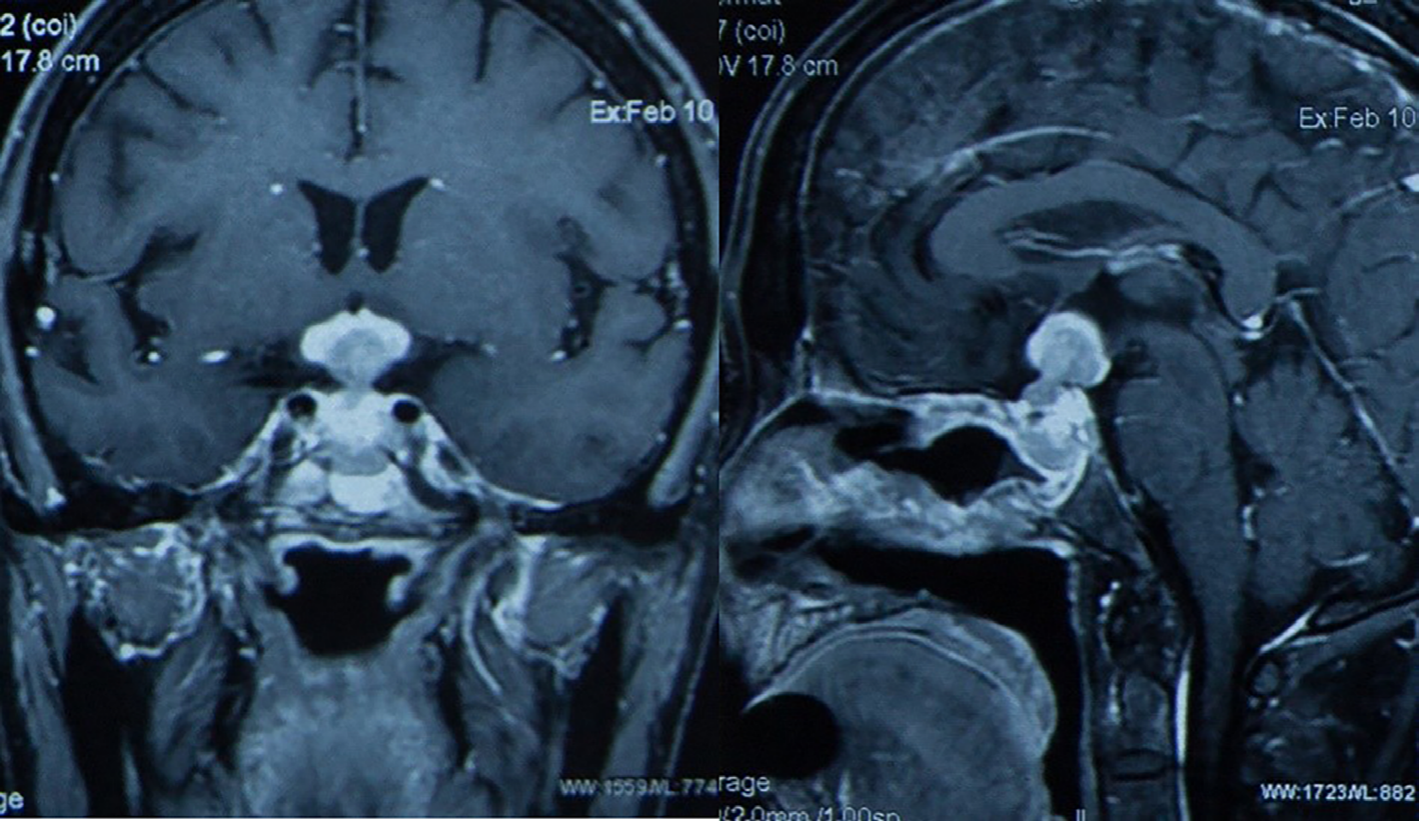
Pituitary MRI at two months from the beginning of chemotherapy demonstrated the tumor (size of about 2 × 1.5 × 3.2 cm) was larger than before without cavernous sinus involving in Case 2.
